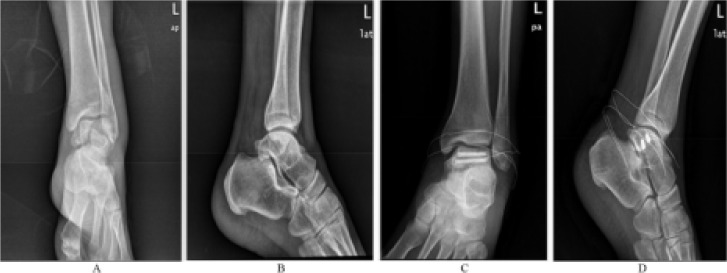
Comparison the results of preoperative and postoperative X-ray examination
